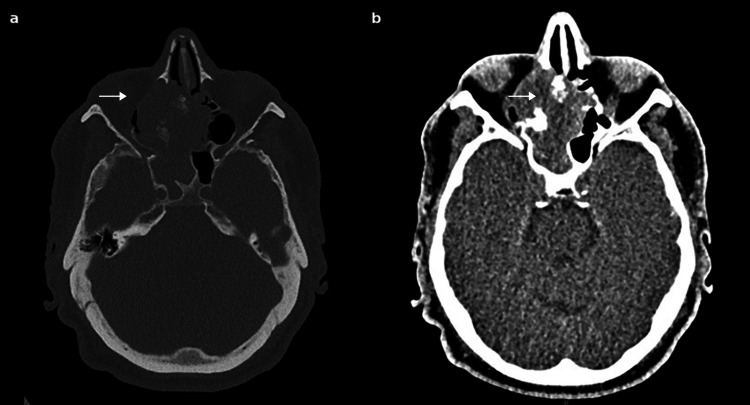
Axial CT scan findings Axial CT scan of the sinonasal cavities in bone window (a) and soft tissue window (b) showing an expansile mass filling the right ethmoidal sinus with extension into adjacent structures (arrows).
